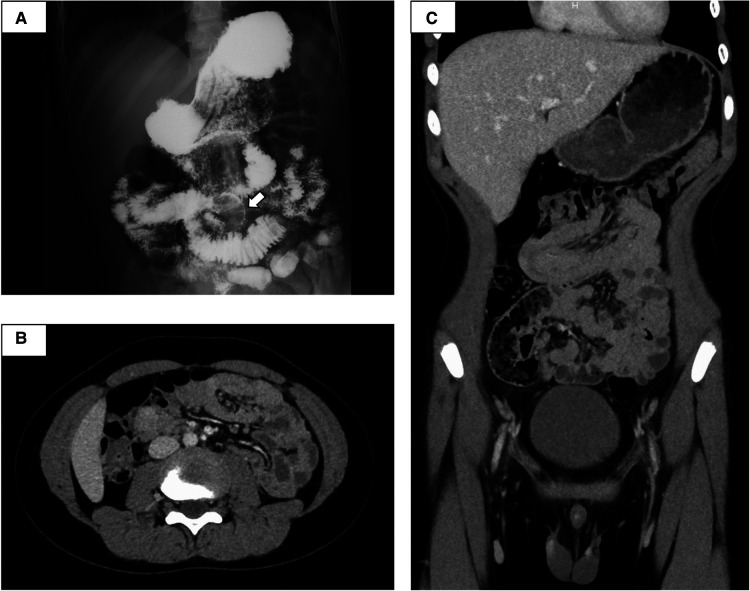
Small intestine fluoroscopy revealed a polyp in the jejunum (A), and abdominal computed tomography showed two polyps and intussusception (B,C). White arrow, polyp.
